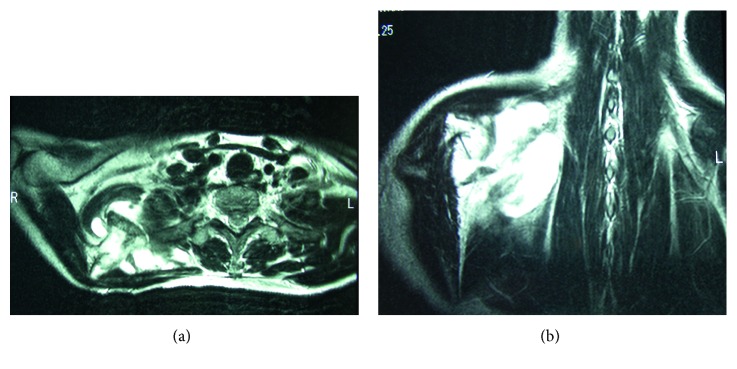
Preoperative T2-weighted magnetic resonance imaging. (a) Axial view demonstrated the cystic lesion containing a large amount of fluid that surrounded the osseous tumor. (b) Coronal view revealed that the cystic lesion spread over the upper two-thirds of the scapula.
